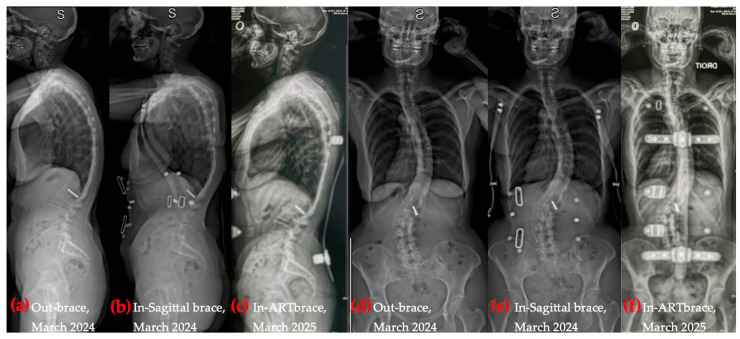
Coronal and sagittal radiographic progression over time, with and without bracing. (a) Out-of-brace, sagittal X-ray; (b) In sagittal brace, sagittal X-ray; (c) In ARTbrace, sagittal X-ray; (d) Out-of-brace, coronal X-ray; (e) In sagittal brace, coronal X-ray; (f) In ARTbrace, coronal X-ray.
